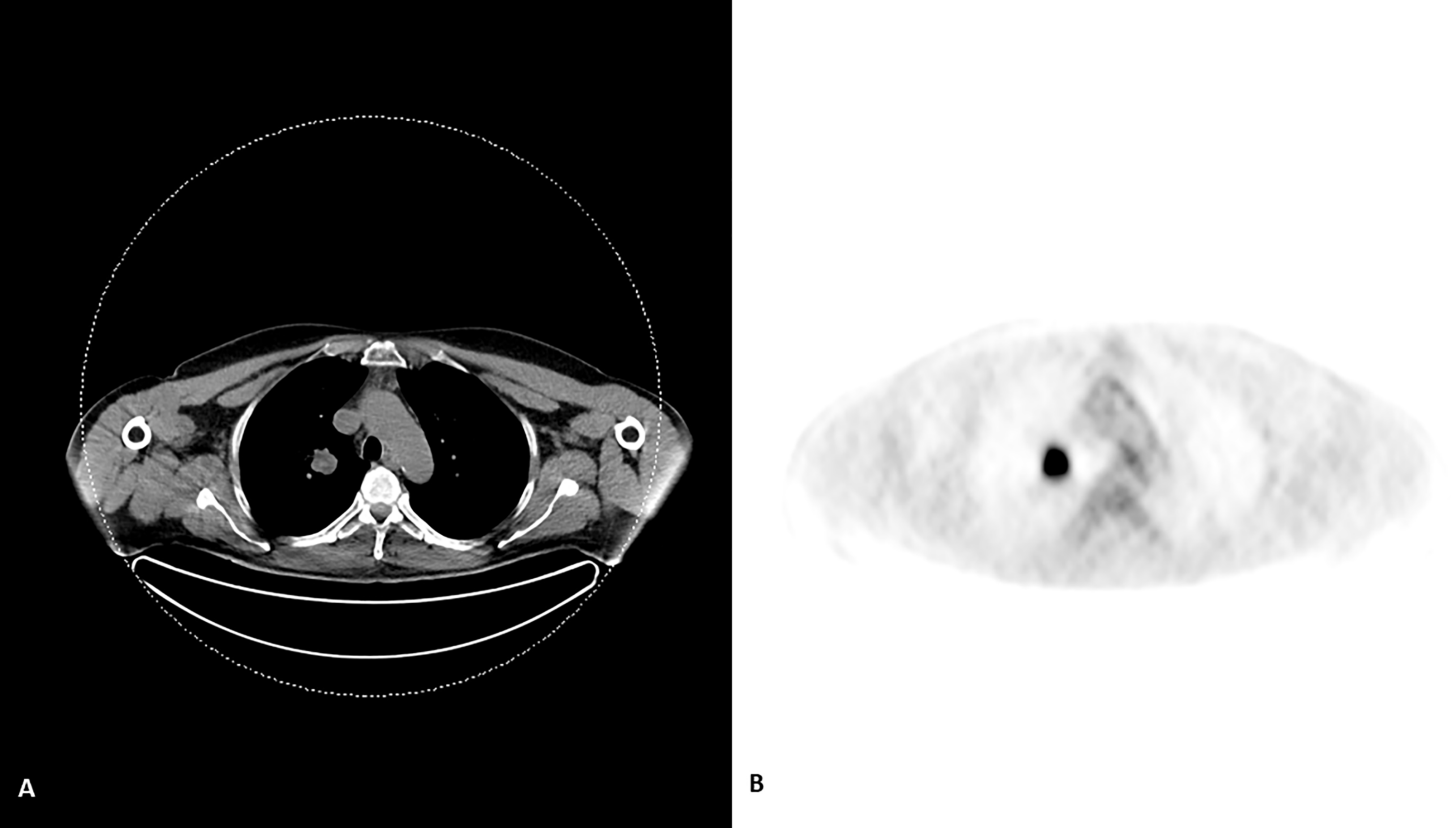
An evident lesion in the upper lobe of the right lung on CT scan (A) with an avid FDG uptake (B) of the compatible with cancer on PET scan.
